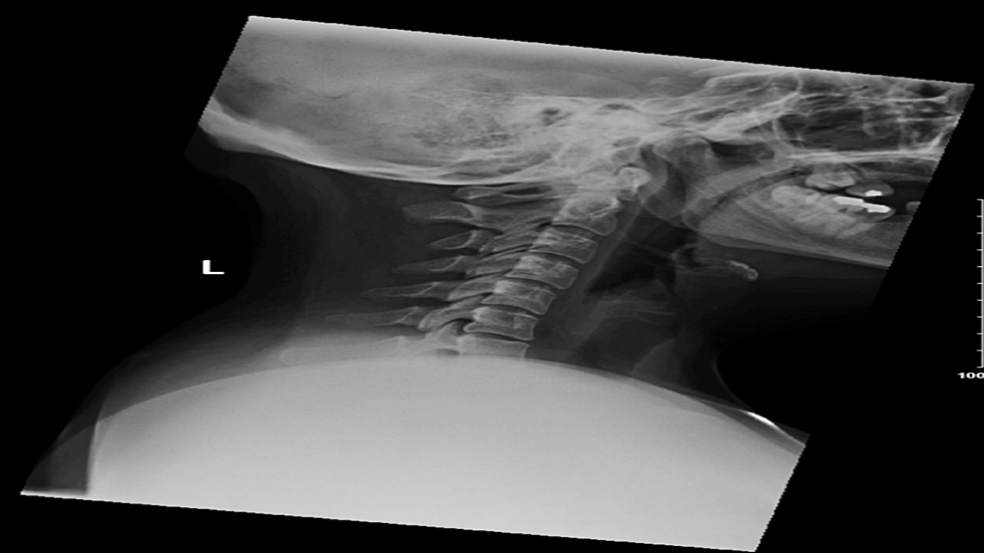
Lateral view of cervical spine taken on x-ray for the patient X-ray taken at the initial presentation of the patient to the emergency department at the onset of symptoms; no evidence of fractures or gross abnormalities to be noted.
